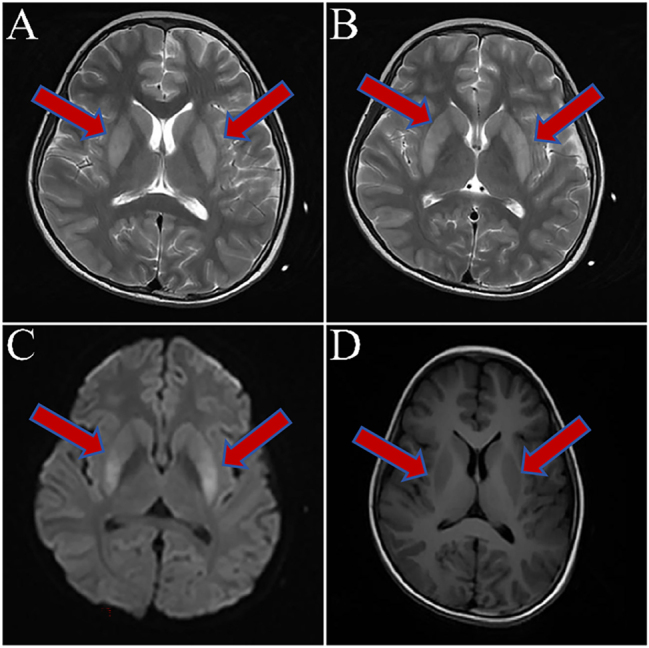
MRI showed symmetrical abnormal signals in the bilateral basal ganglia region. A–D demonstrated a nodular high signal in the both sides of the basal ganglia (white arrows) on T2WI (A), FLAIR (B), and DWI (C) while presented low signal on T1WI (D).
